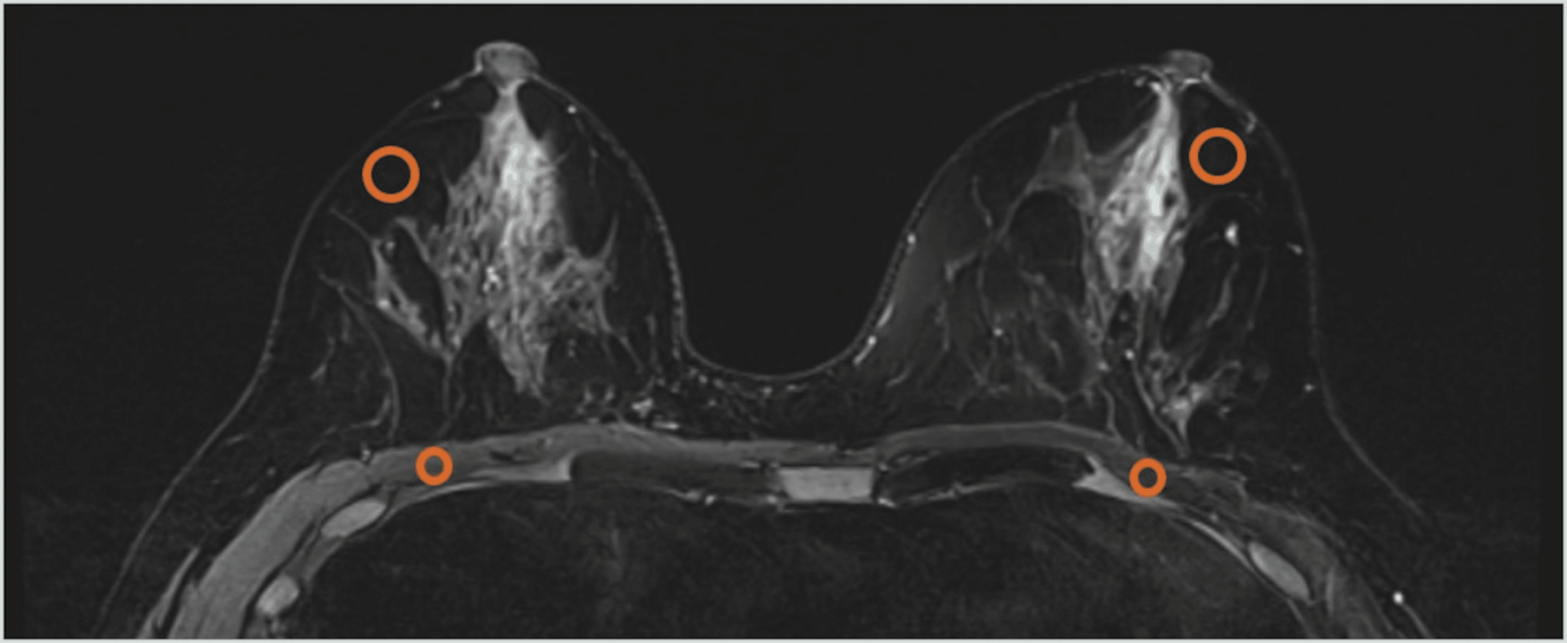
Representative placement of regions of interest for signal-to-noise ratio and contrast ratio on fat-suppressed T2-weighted breast MRI Orange circles indicate the circular regions of interest (ROIs). ROIs were placed within the bilateral pectoralis major muscles and within homogeneous fat regions in the fat-suppressed image. ROIs were positioned to avoid artifacts and large vessels and were copied to the corresponding locations on the paired reconstruction.
